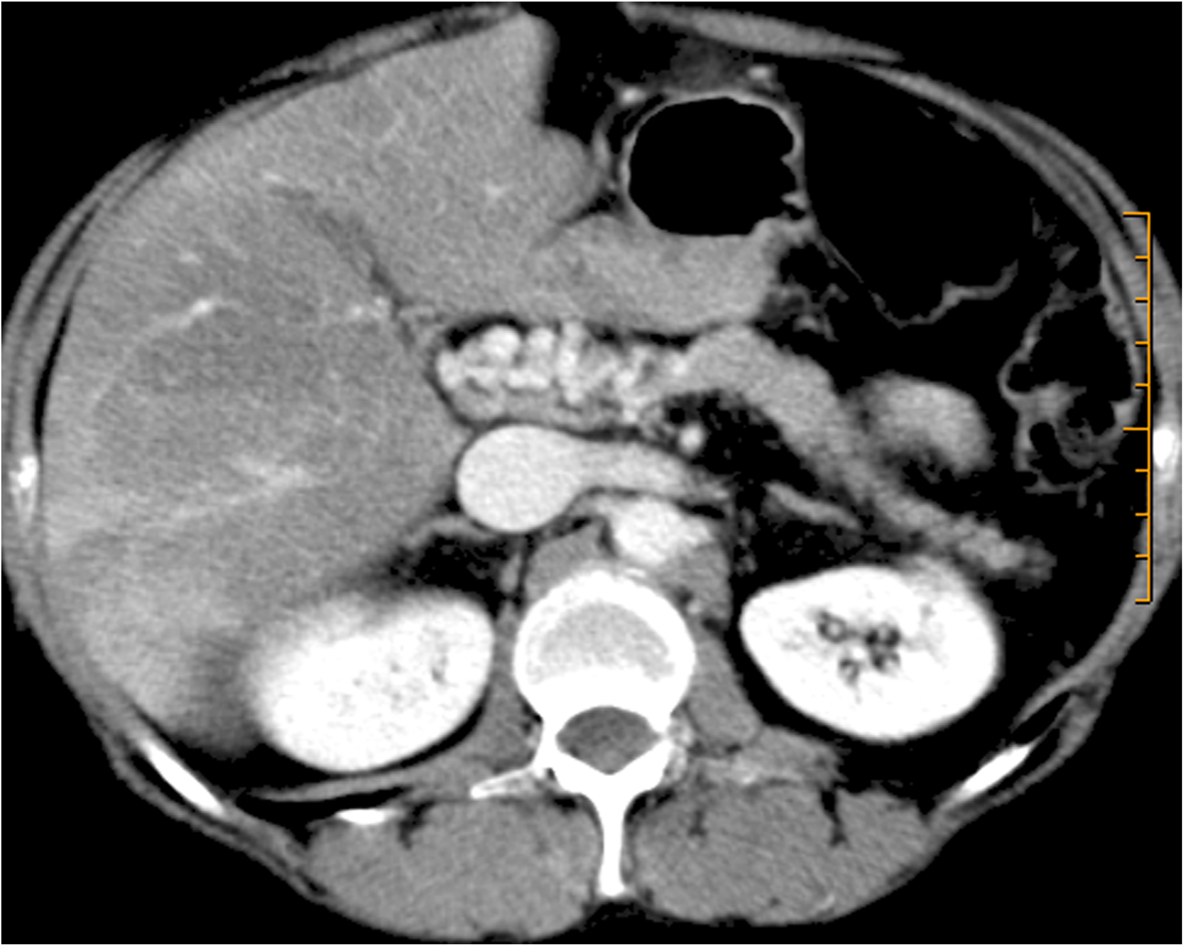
Cavernomatous transformation of PV. Late arterial phase CT shows absence of a well-defined PV and multiple enhancing veins instead, representing portoportal collateral vessels—portal cavernoma. Note the THED associated with cavernoma
